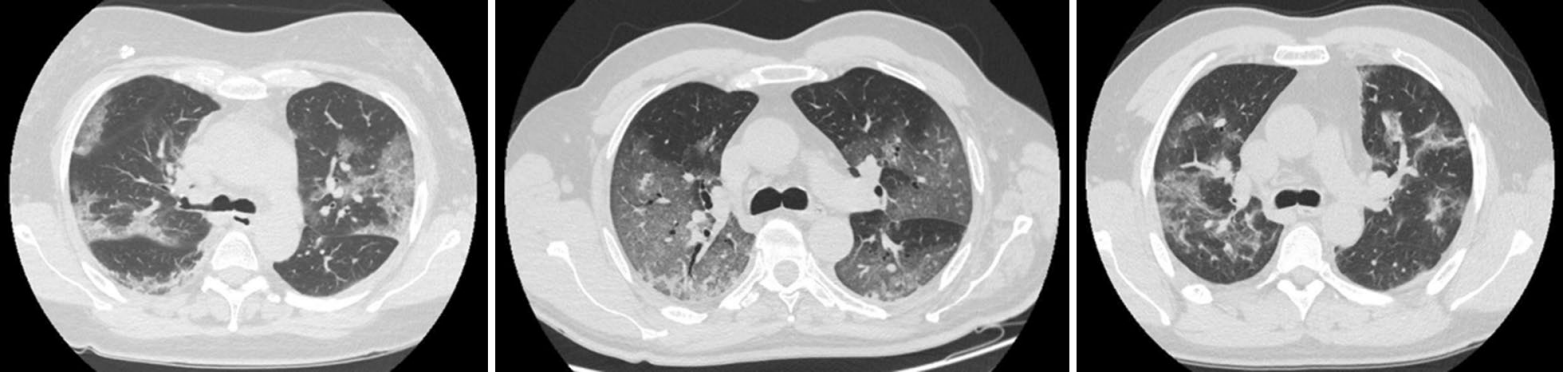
Lung Computed Tomography. Lung Computed Tomography images (at the level of the carina), extracted from lung CT of three different patients, representative of the ARDS severity of the study population
